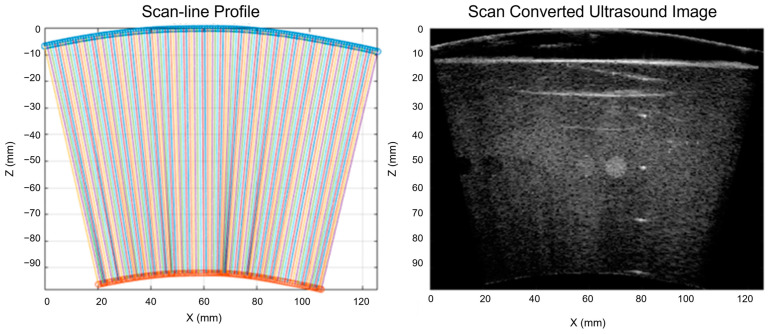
Profile of each scan-line and the corresponding scan converted ultrasound image.
